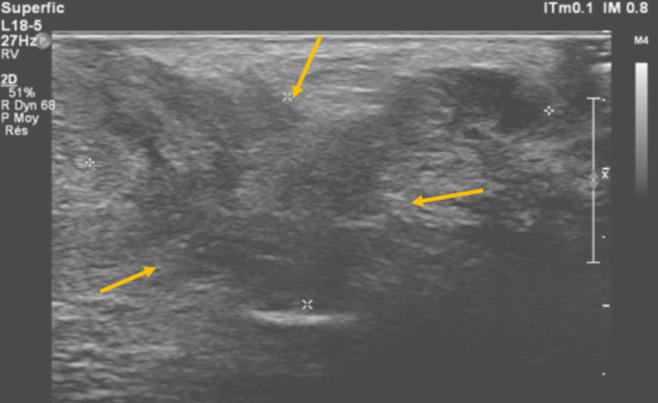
transperineal ultrasound revealed a heterogeneous hypoechoic mass in the right perineum, with retractile and irregular contours measuring 32x15mm (yellow arrows)
